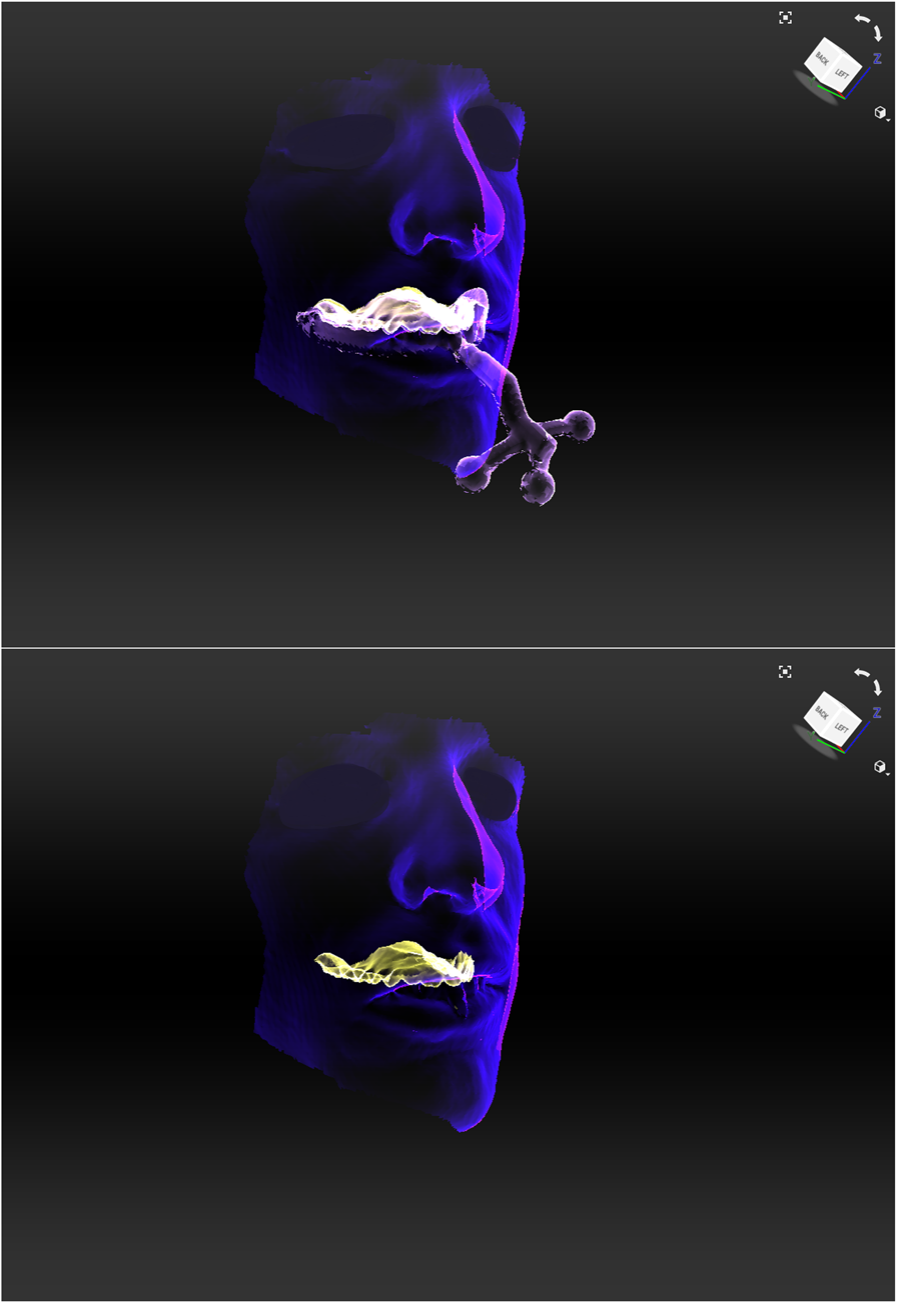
Alignment of the facial contours (blue) and the dentoalveolar impression. X-ray mode makes it possible to see the actual position of the dentoalveolar arch (yellow) in relation to facial contours
